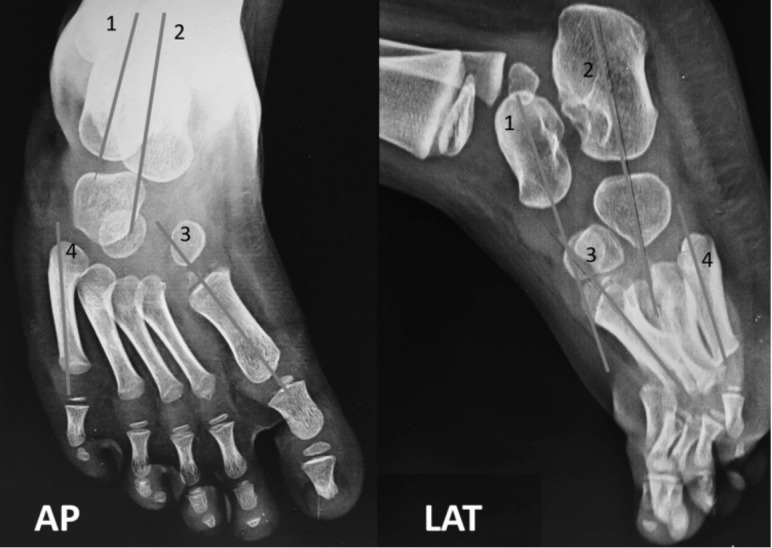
Anteroposterior and lateral image of the foot showing the radiographic parameters used. (1) Axis of talus. (2) Axis of calcaneum. (3) Axis of 1st metatarsal. (4) Axis of 5th metatarsal. Corresponding angles between these axes were measured.
